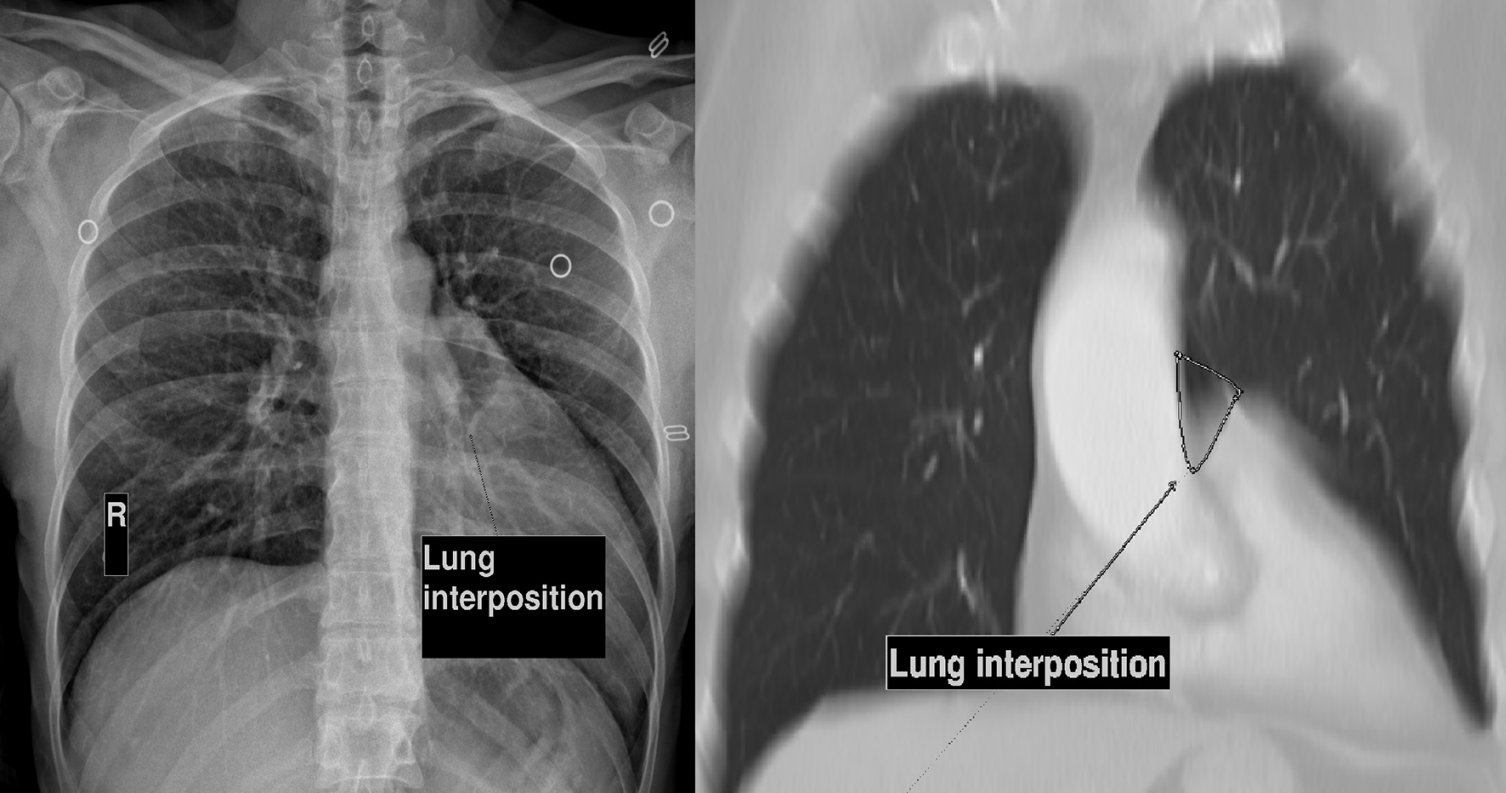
(a). Chest X-ray shows leftward position of the heart (Snoopy sign) and a hyperlucency between the aorta and main pulmonary artery (b). MIP reconstruction documents lung between the aorta and main pulmonary artery, region commonly lung-free. MIP, maximum intensity projection.
